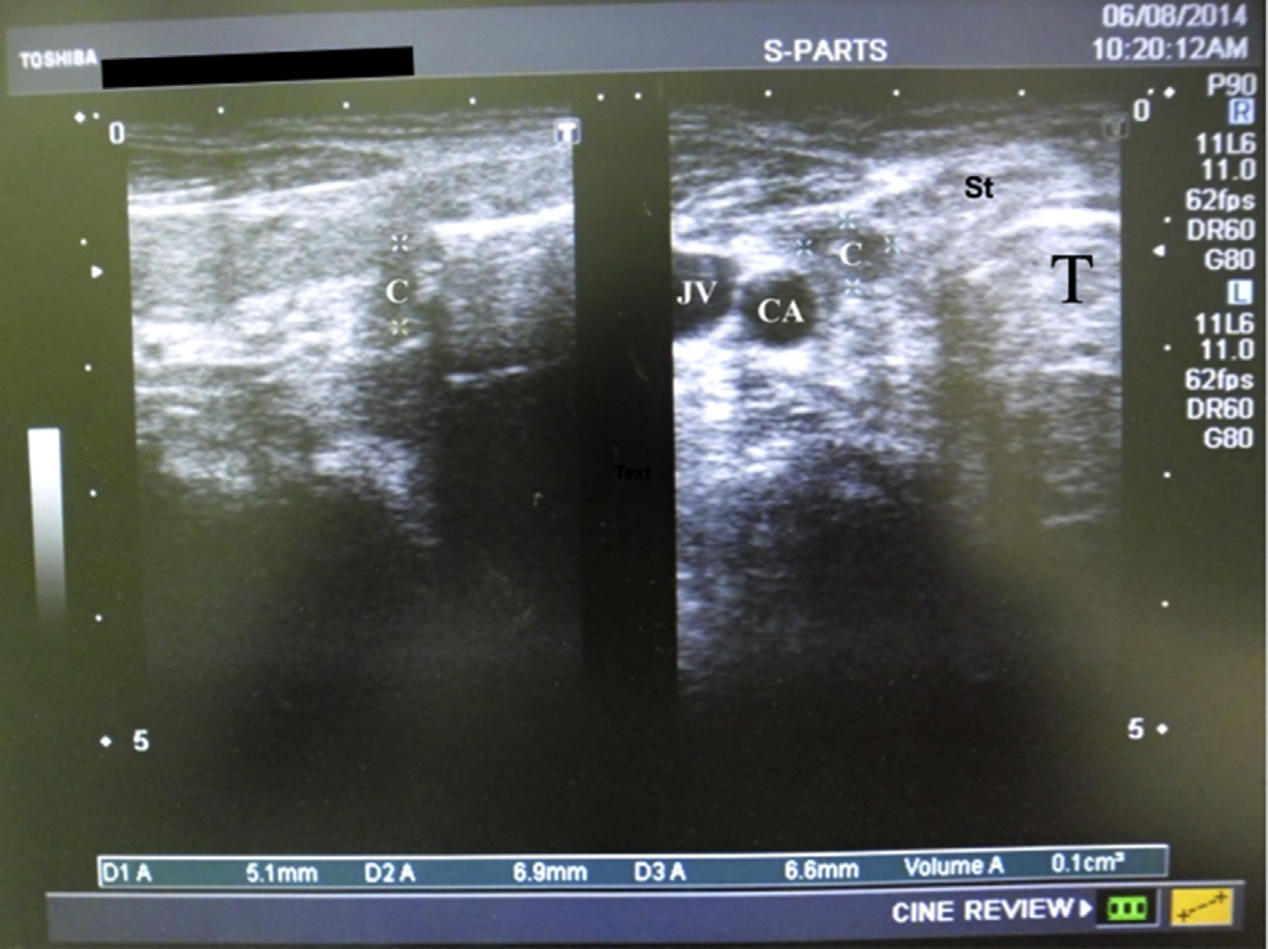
Ultrasound image of the neck with deep collection 2 days postoperative. Right side shows cross-section, left side shows saggital section.
